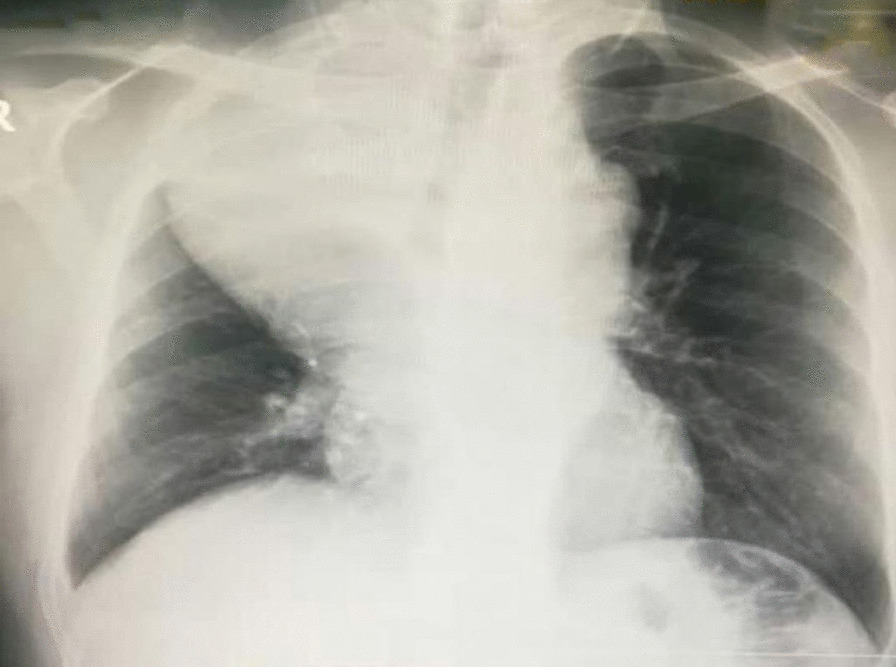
Presurgical Chest X-Ray showing a density occupying the right lung, suggestive of a possible mass
